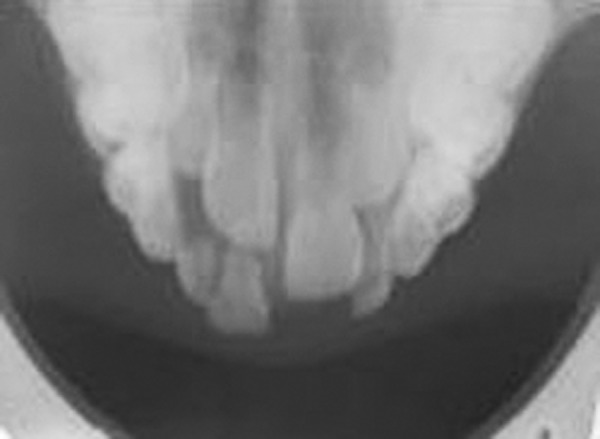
Intraoral occlusal radiograph showing radiolucency in relation to the apices of left deciduous incisors
